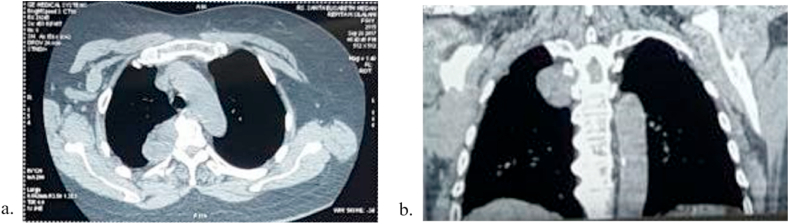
Follow-up contrast-enhanced CT in 12th January 2018 (a) axial view (b) coronal view. Figures (a) and (b) showed a lobulated mass in the right medial posterior intrathoracic mass attached to the 3rd-5th vertebrae body with size 5x3.2 × 2.8cm and without connection to the spinal canal. There is no destruction of vertebrae bone. Compared with recent Chest CT, there was no significant gain of mass.
